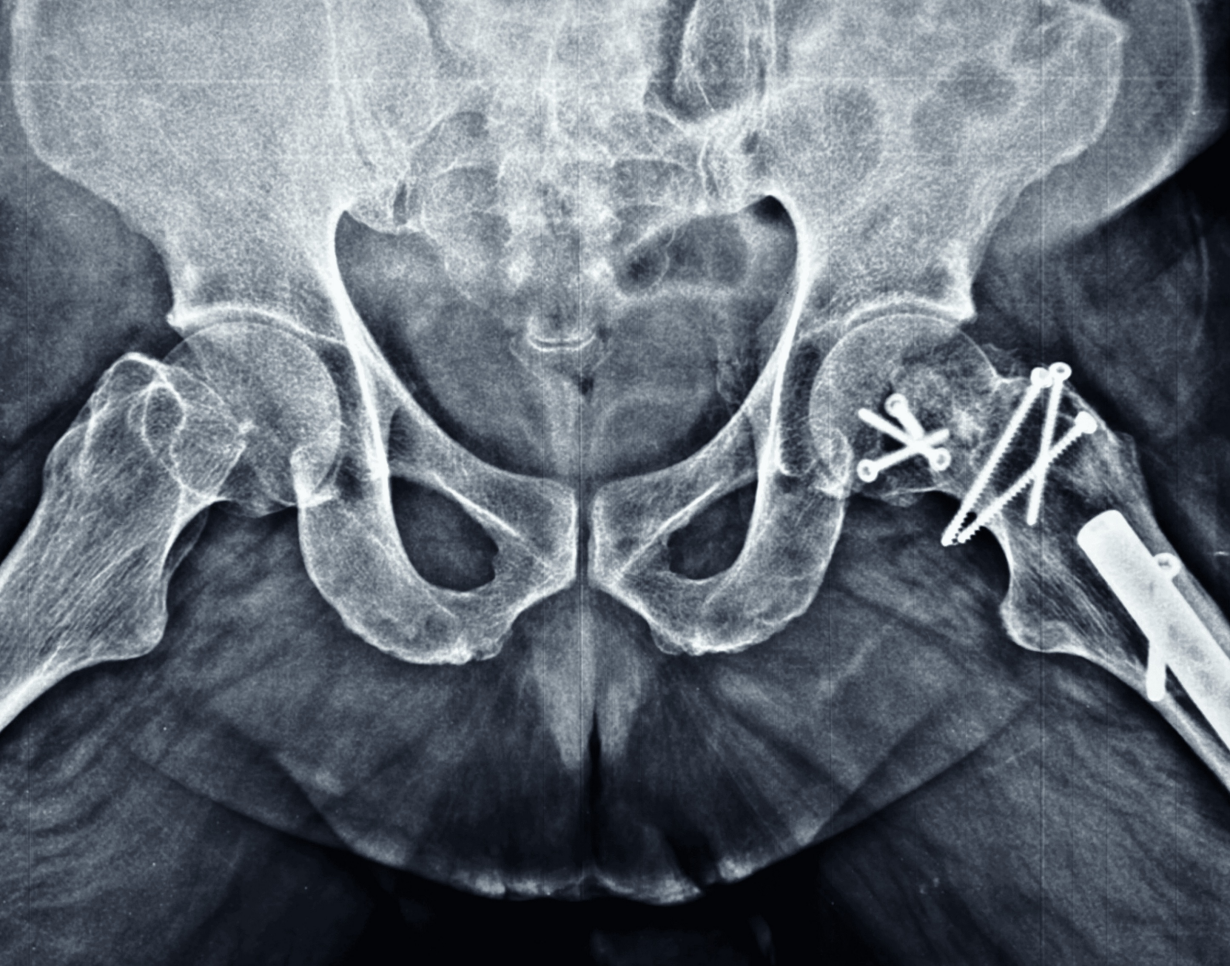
At 96 months follow-up, X-ray of both hips frog lateral view – no signs of avascular necrosis (AVN) or post-traumatic arthritis (PTA)
